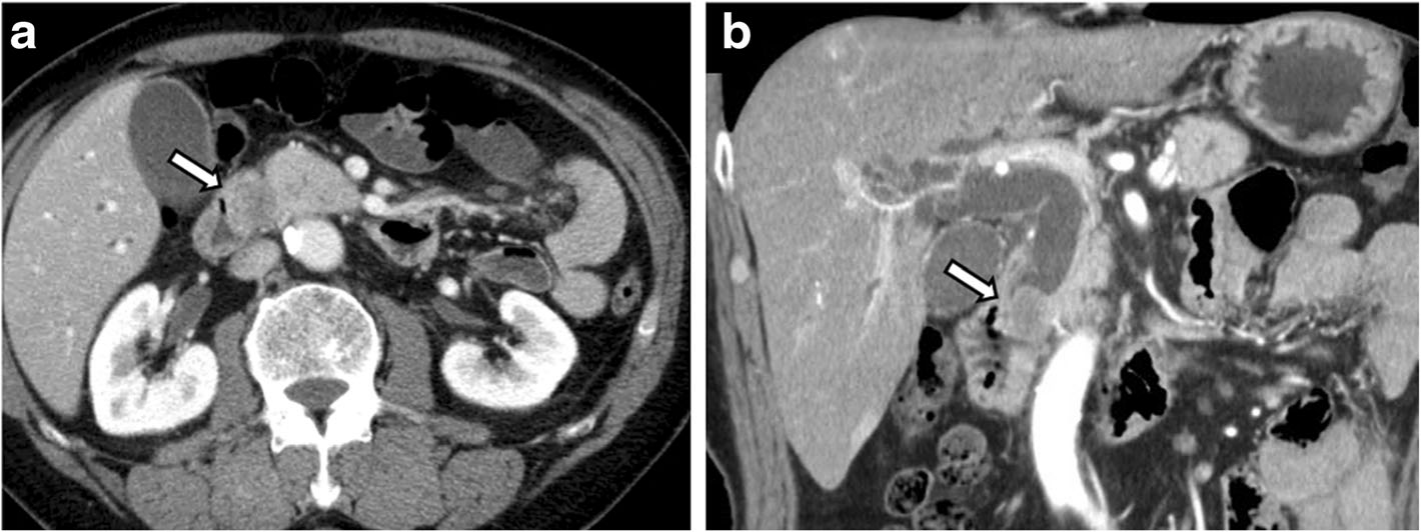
Abdominal CT. Axial (a) and coronal (b) images show a 3-cm tumor in the ampulla on Vater (*white arrow*) and dilatation of the common bile duct
